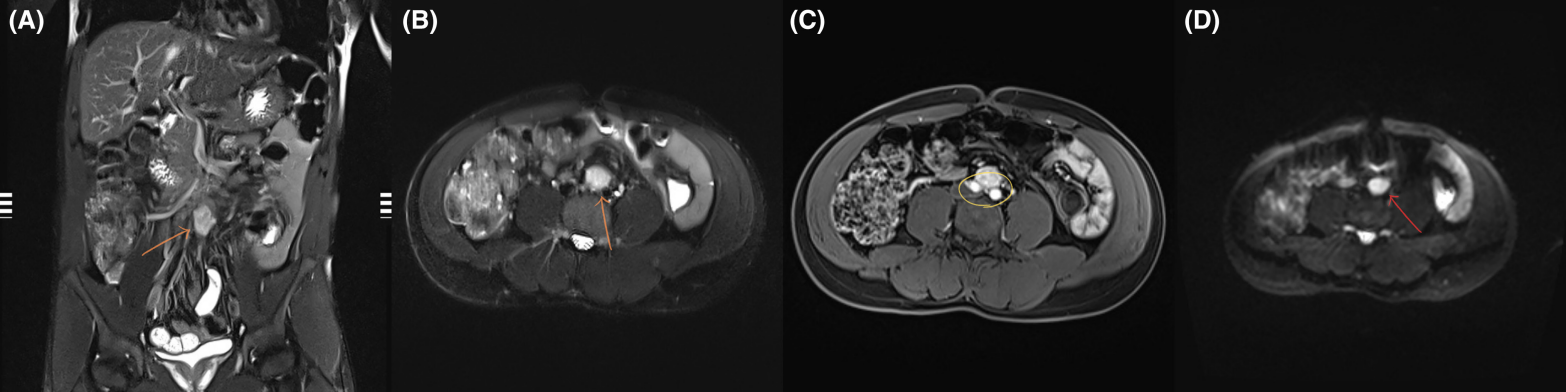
Coronal T2 fat‐saturated (A), axial T2 fat‐saturated (B), gadolinium‐enhanced axial T1 fat‐saturated (C), and DWI (D) MRI images showcasing the 21 × 21 × 29 mm lesion at the aortic bifurcation. The lesion demonstrates increased T2 signal (orange arrow), heterogeneous enhancement patterns (yellow circle), and restricted diffusion (red arrow).
